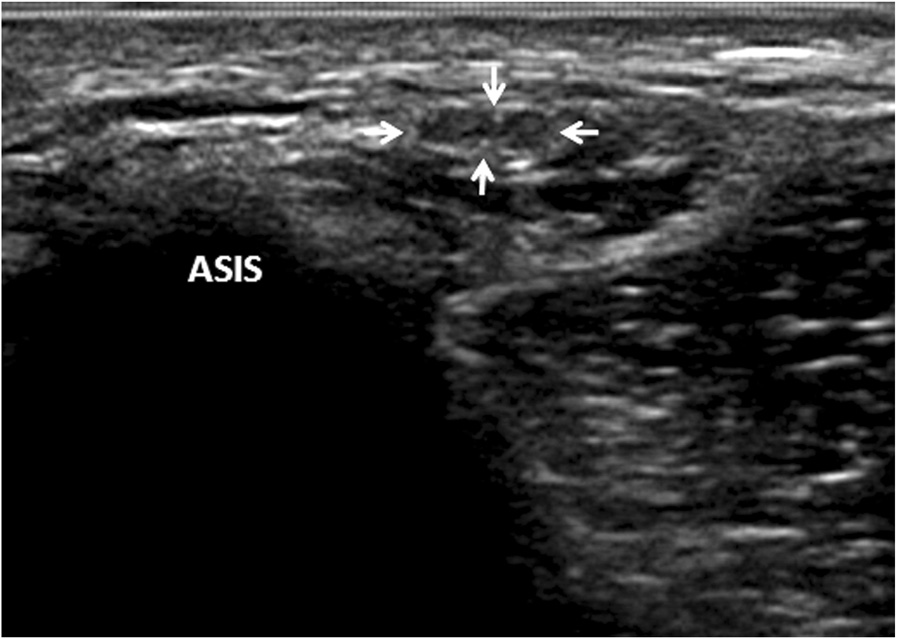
Transverse ultrasound image shows a neuroma (arrow) of the LFCN. ASIS: anterior superior iliac spine.
